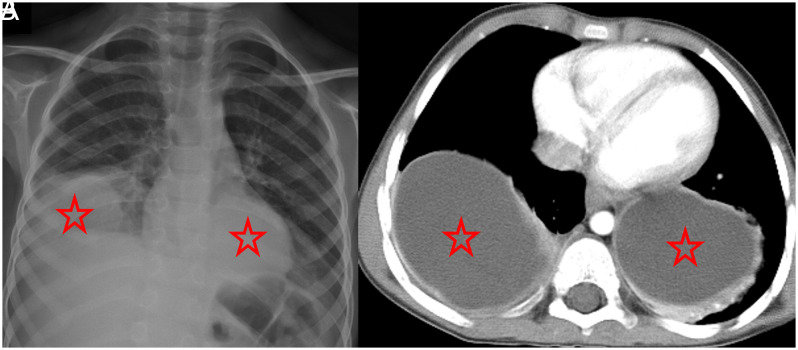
Posteroanterior chest radiograph (A) and axial chest CT (B) of a 5-year-old male patient with intact cysts measuring approximately 75 mm in the right lower lobe and 65 mm in the left lung (asterisks).
